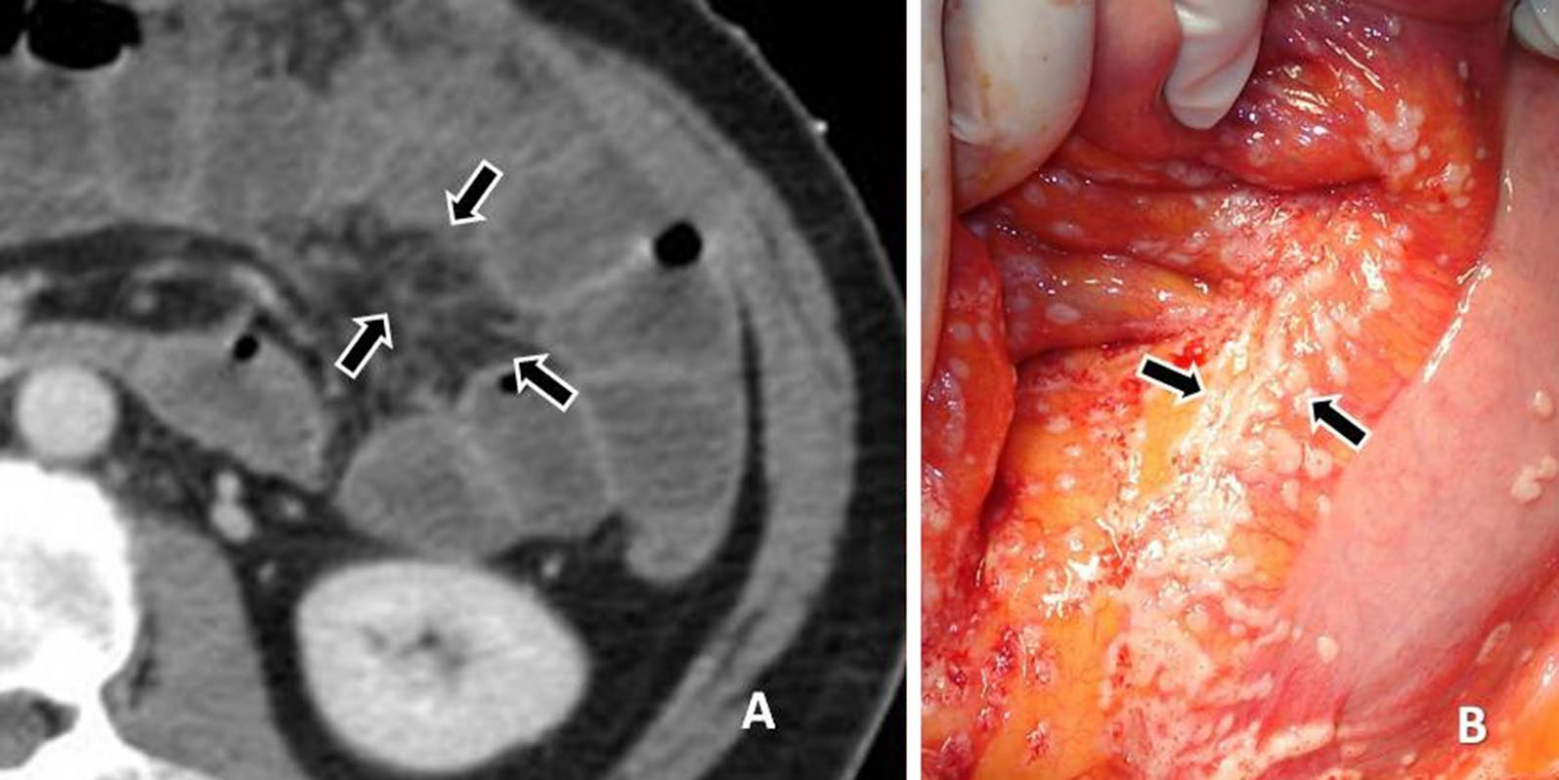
a CT enteroclysis image discloses increased attenuation and stranding of the mesenteric fat (arrows). b At surgery the CT finding is produced by multiple tiny implants on the small bowel mesentery (arrows)
